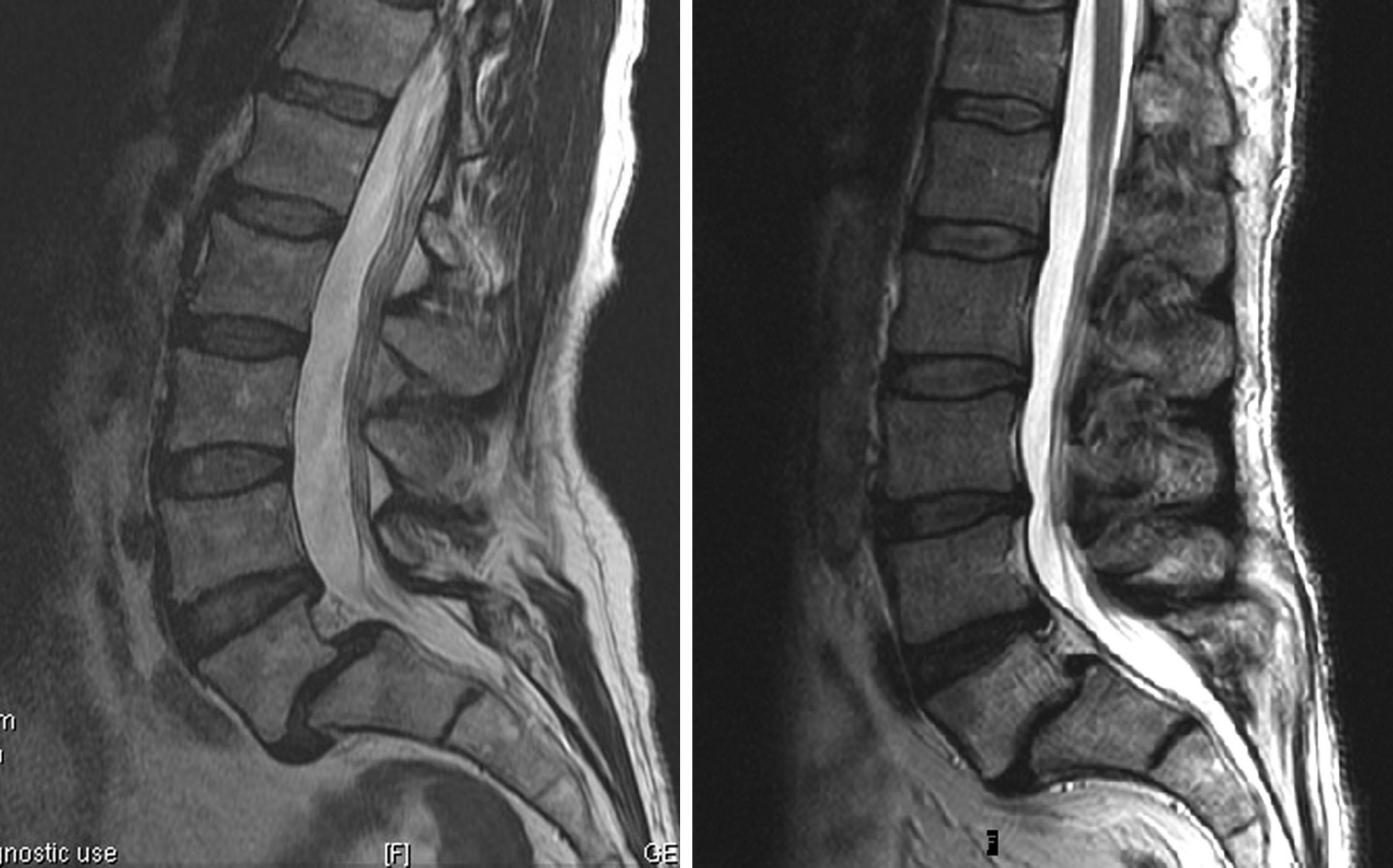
Lumbar midline sagittal T2-weighted magnetic resonance images. Grade 2 L5-S1 spondylolisthesis (case 1, left) and combined grade 2 L5-S1 anterolisthesis and grade 1 L4-5 retrolisthesis (case 3, right) are shown.
